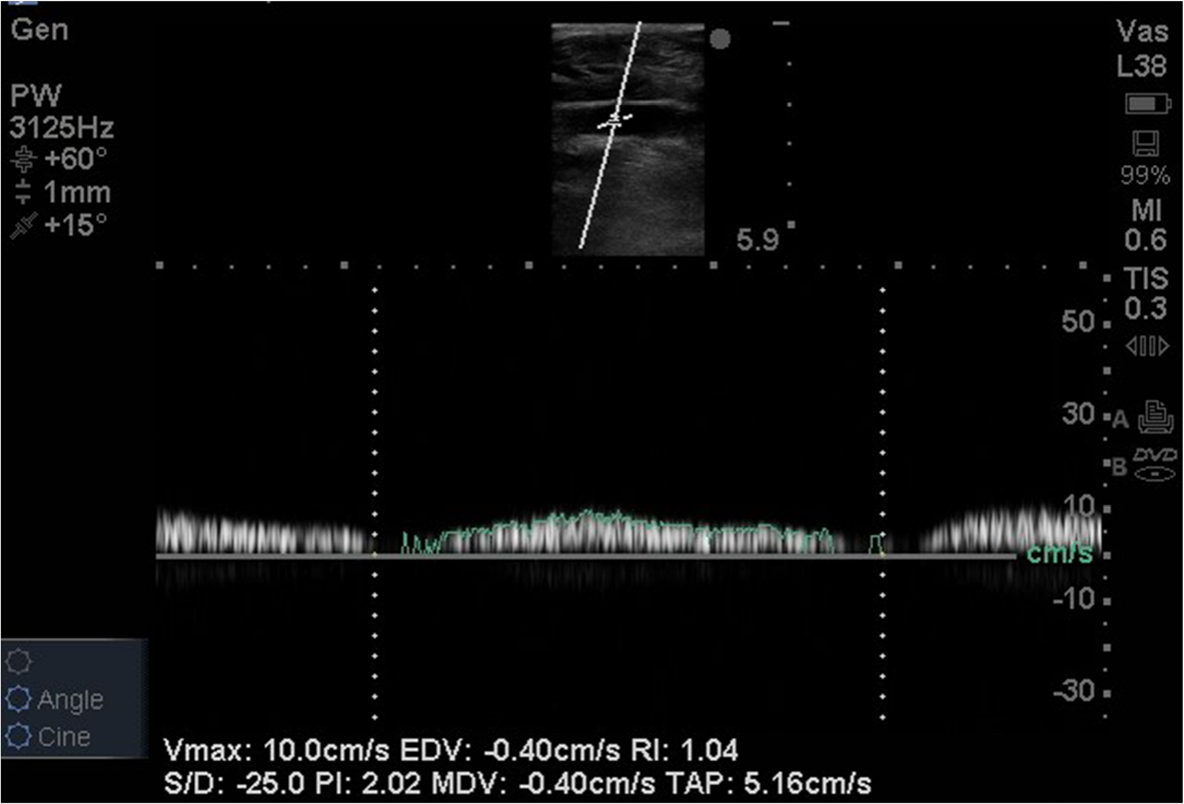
The Doppler sample volume cursor was placed in the center of the vein, and mean blood velocity and maximum blood velocity were calculated
